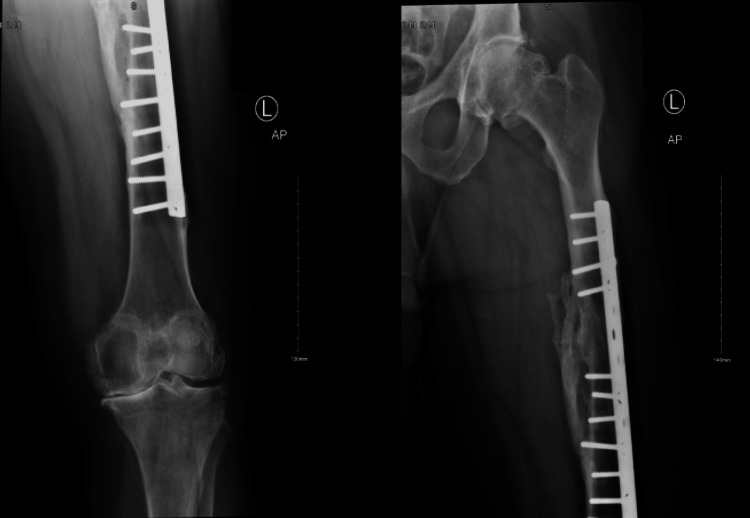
Radiographs showing AP view for left femur fracture plate fixation
